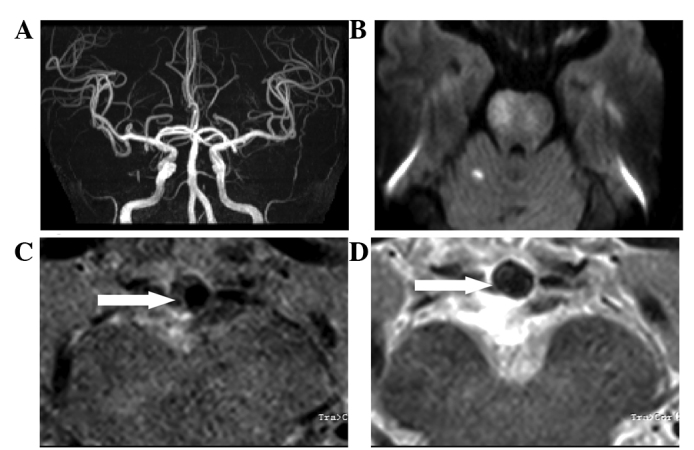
A 60-year-old female presented with an episode of sudden onset left face, arm and leg weakness. (A) MRA revealed an irregular mild BA stenosis close to the pontine paramedian artery. (B) An acute high-intensity infarction was demonstrated on the axial DWI image at the level of the right lateral pontine. (C and D) Cross-sectional T1- and T2-weighted HRMRI at the level of the BA revealed a lipid-rich necrotic eccentric plaque with low or iso-signal density. The arrows represent the position of the lesion. MRA, magnetic resonance angiography; BA, basilar artery; DWI, diffusion-weighted imaging; HRMRI, high-resolution magnetic resonance imaging.
